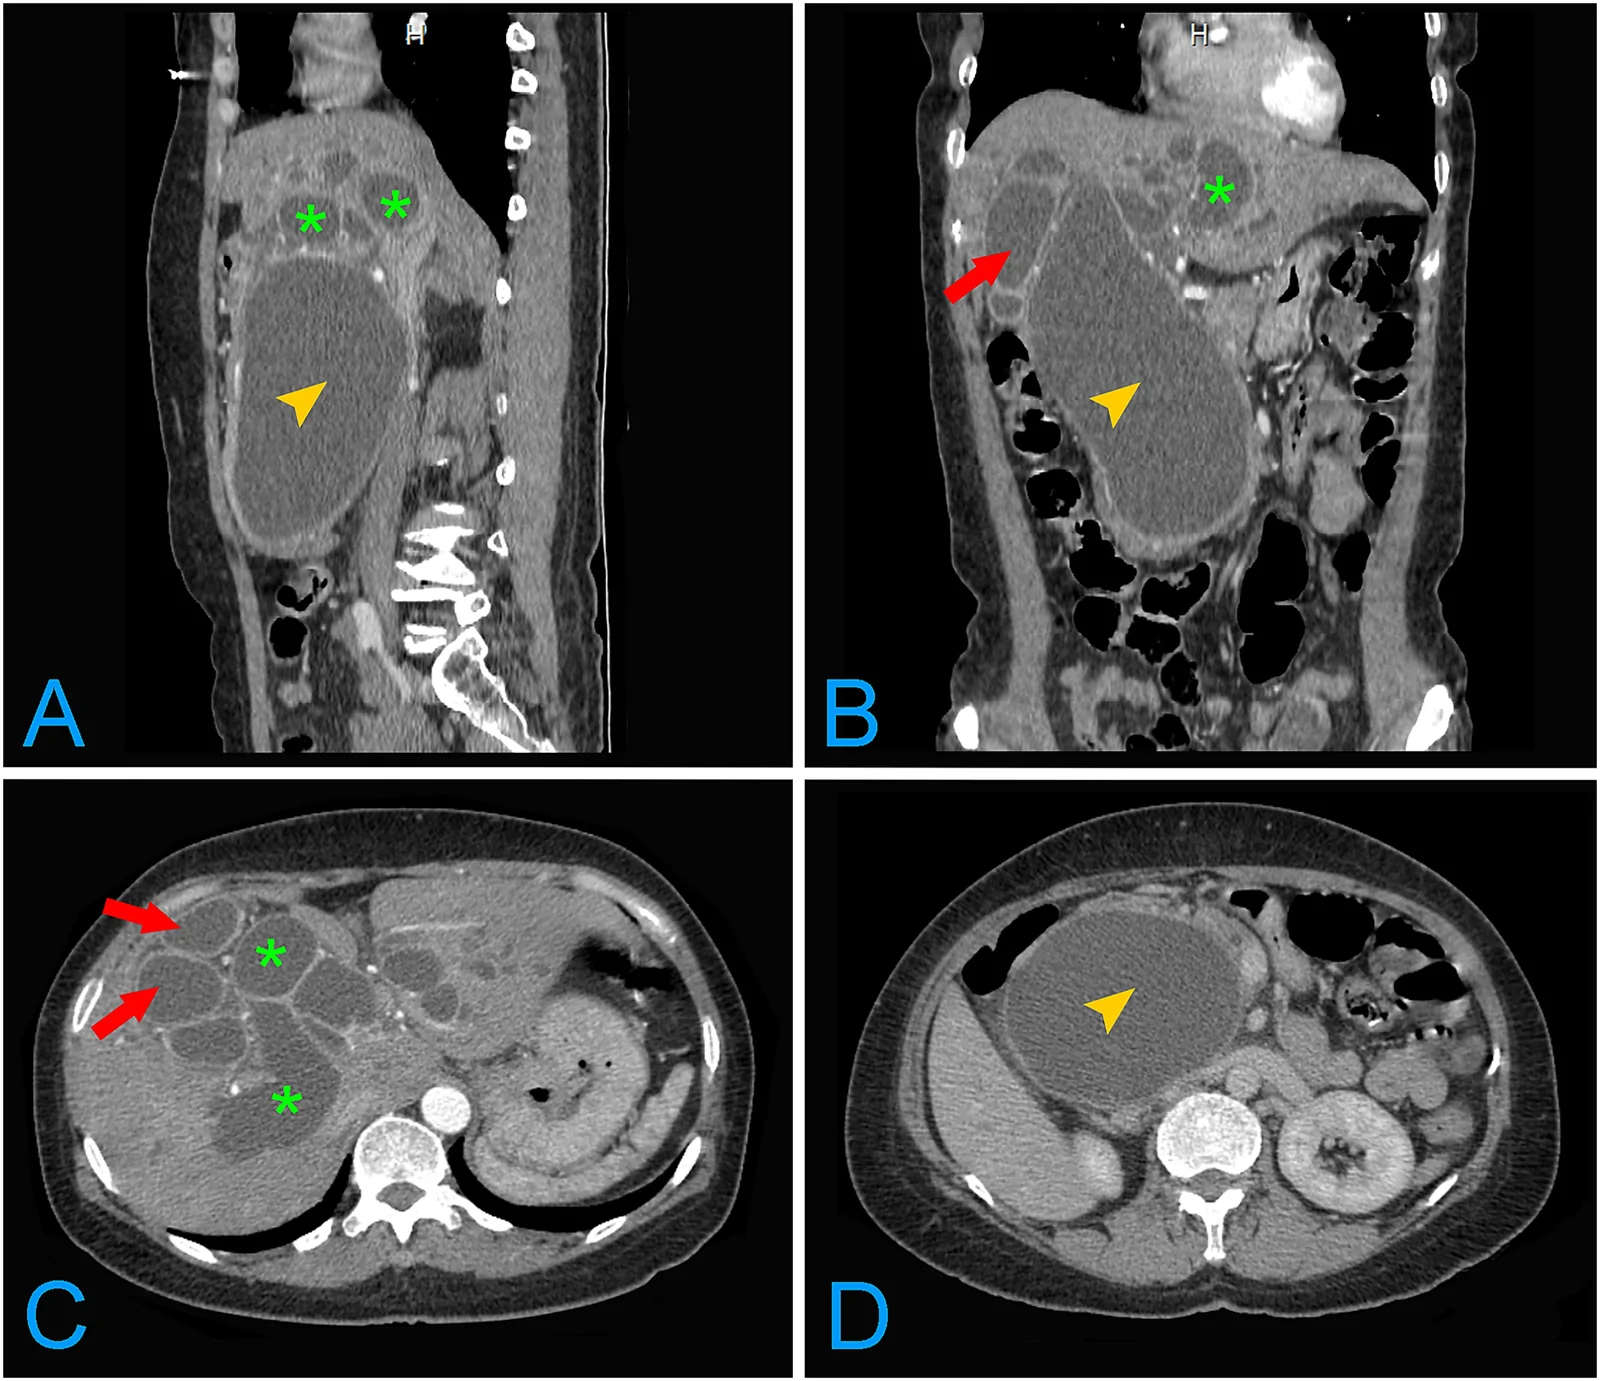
Contrast-enhanced CT. Sagittal (A), coronal (B), and axial (C, D) images demonstrate a giant cystic dilatation of the extrahepatic bile duct (arrowheads), marked intrahepatic biliary dilatation (asterisks), and a distended gallbladder (arrows), compatible with Todani type IVa choledochal cyst.
